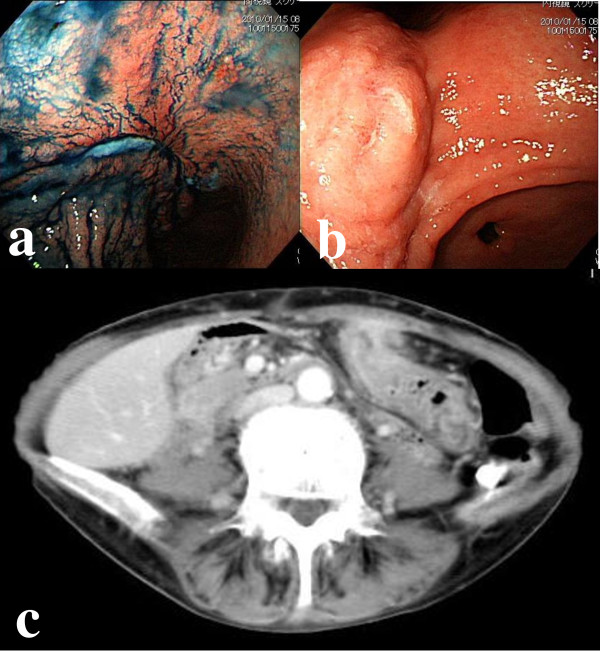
Gastrointestinal endoscopic examination and Computed tomography. a. A gastrointestinal endoscopic examination was performed and disclosed an ulcerated lesion in the lesser curvature of the gastric corpus located at 7 cm from the esophagogastric junction, which squashed and isolated the gastric folds from the rest of the stomach. b. An elevated lesion that appeared to be a submucosal tumor (SMT), which was suspected of being an advanced gastric cancer, was detected on the anal side of the ulcerated lesion. c. Computed tomography (CT) revealed thickening of the gastric wall and findings indicative of abdominal wall invasion.
